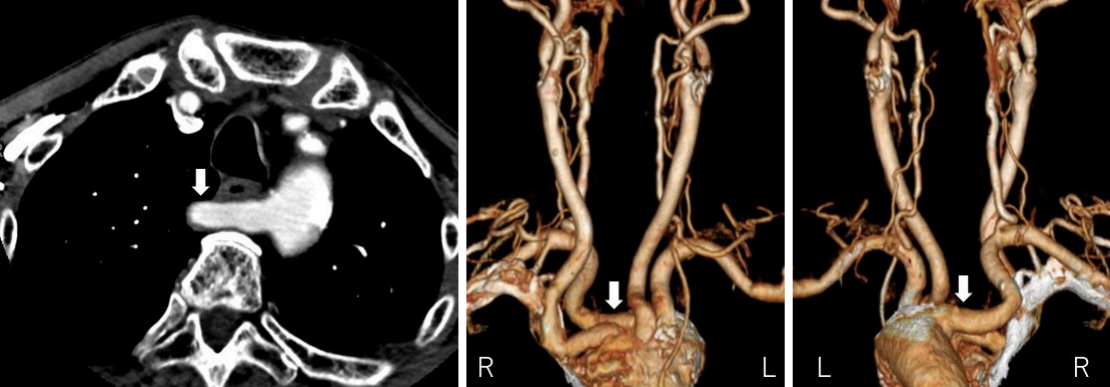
Preoperative three-dimensional computed tomography angiography showing an aberrant right subclavian artery with a retroesophageal course originating from the most distal branch of the aortic arch (arrow).
